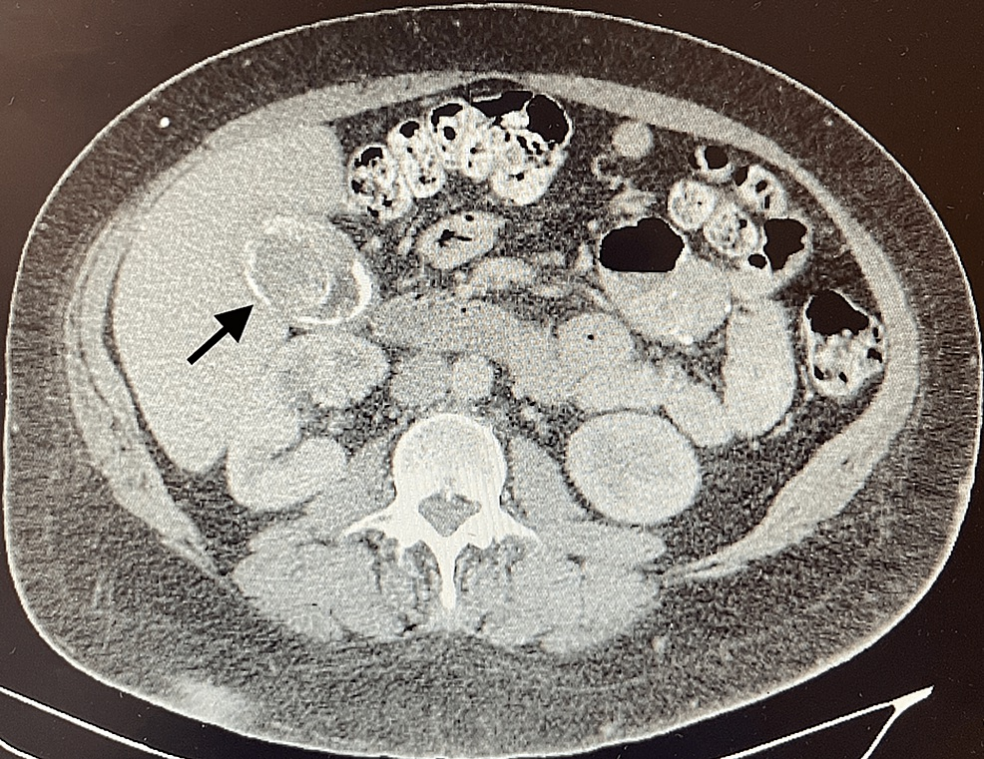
CT of the abdomen and pelvis with intravenous contrast showing large, partially calcified gallstones, essentially filling the entire gallbladder lumen
